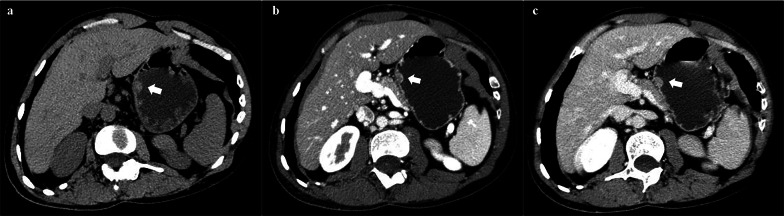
34-year-old man with GCP accompanied by adenocarcinoma. a Axial unenhanced CT reveals an iso-attenuating hemisphere mass (*arrow*) in the lesser curvature of gastric body. b Axial arterial phase CT shows the obvious rim-like enhancement with central low attenuation of this mass (*arrow*). c Axial venous phase CT shows mild enhanced mass (*arrow*). The enhancement area of this mass is gradually expanding compared to the rim-like enhancement in arterial phase
